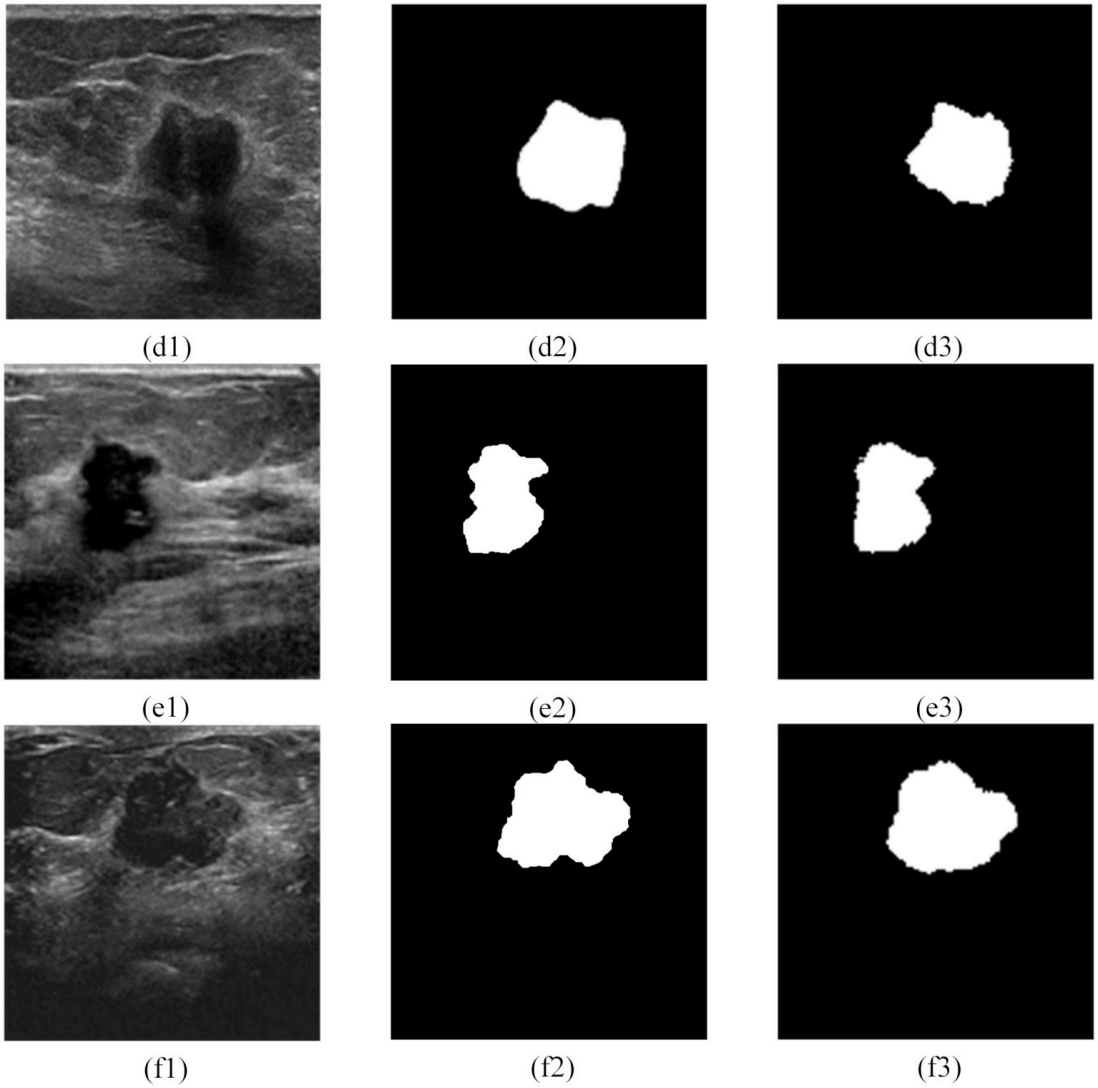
Ultrasound breast tumor segmentation based on the RDAU-NET model. Here(d1)was obtained from Dataset B [13] and (e1) and (f1) were acquired from the Imaging Department of the First Affiliated Hospital of Shantou University. Also(d2), (e2) and (f2) are gold standard and (d3), (e3), (f3) are the results of the RDAU-NET model segmentation.
